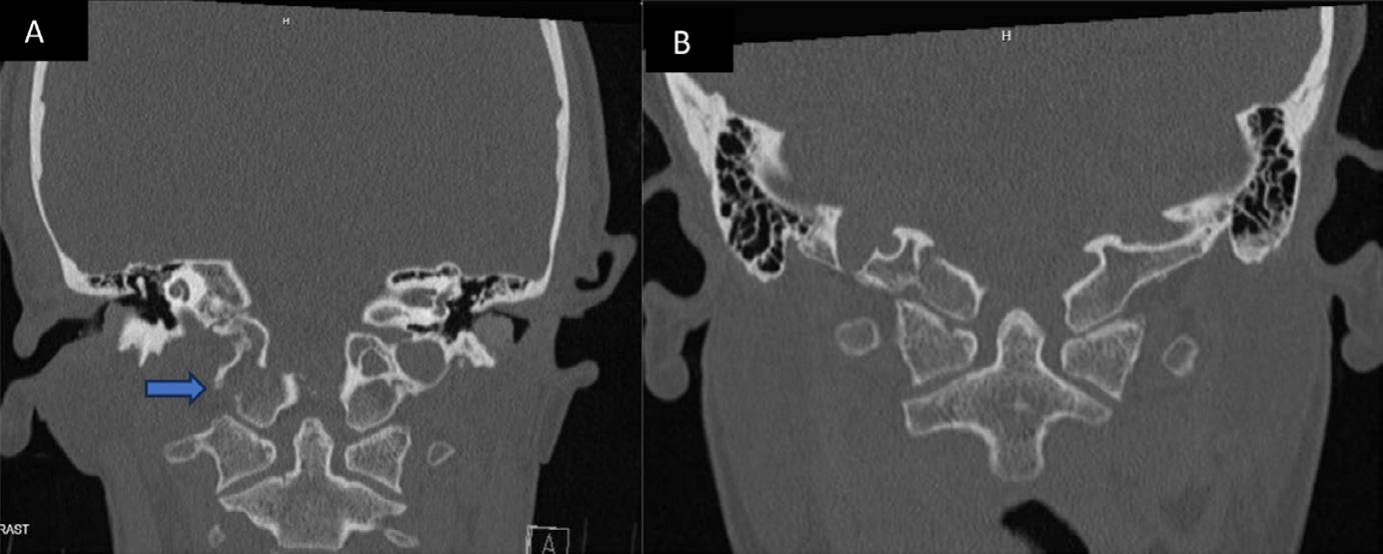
A and B non‐contrast CT maxillofacial (coronal view) showing initial avulsion type fracture through right occipital condyle, and 3 months follow up CT in a similar plane showing healed fracture.
